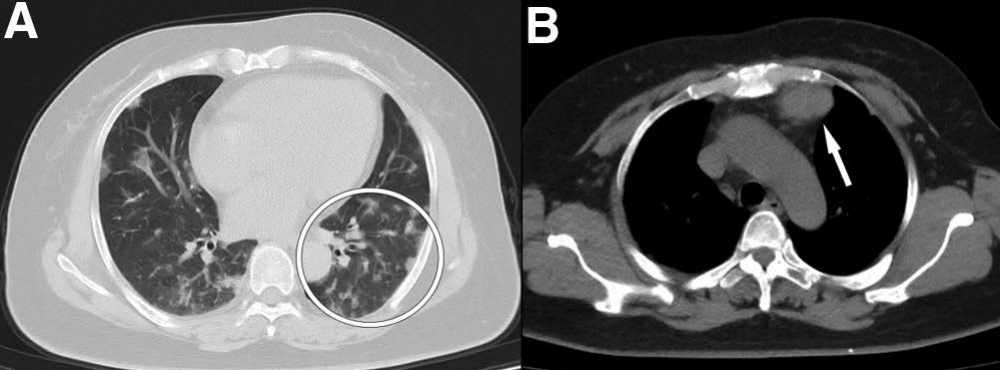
Chest CT imaging obtained 1 wk before admission. Axial images show bilateral multiple patchy high-density shadows and fibrous foci (A, oval) and space occupying lesions in mediastinum (B). CT = computed tomography.
